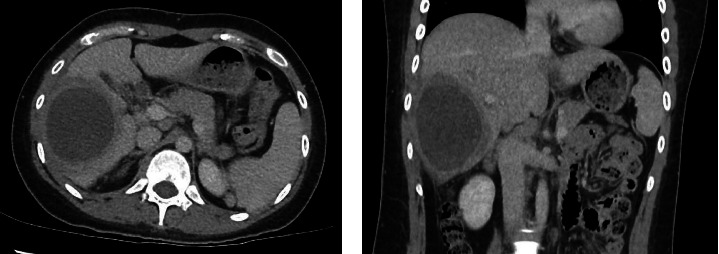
A repeat contrast-enhanced CT scan 11 weeks from the initial scan revealed a significantly smaller, unilocular hepatic lesion measuring 6.2 × 5.5 cm. The lesion had a smooth-enhancing rim surrounded by an outer rim of thick perilesional oedema, giving a ‘double-rim' appearance. (a) Transverse plane. (b) Coronal plane.
